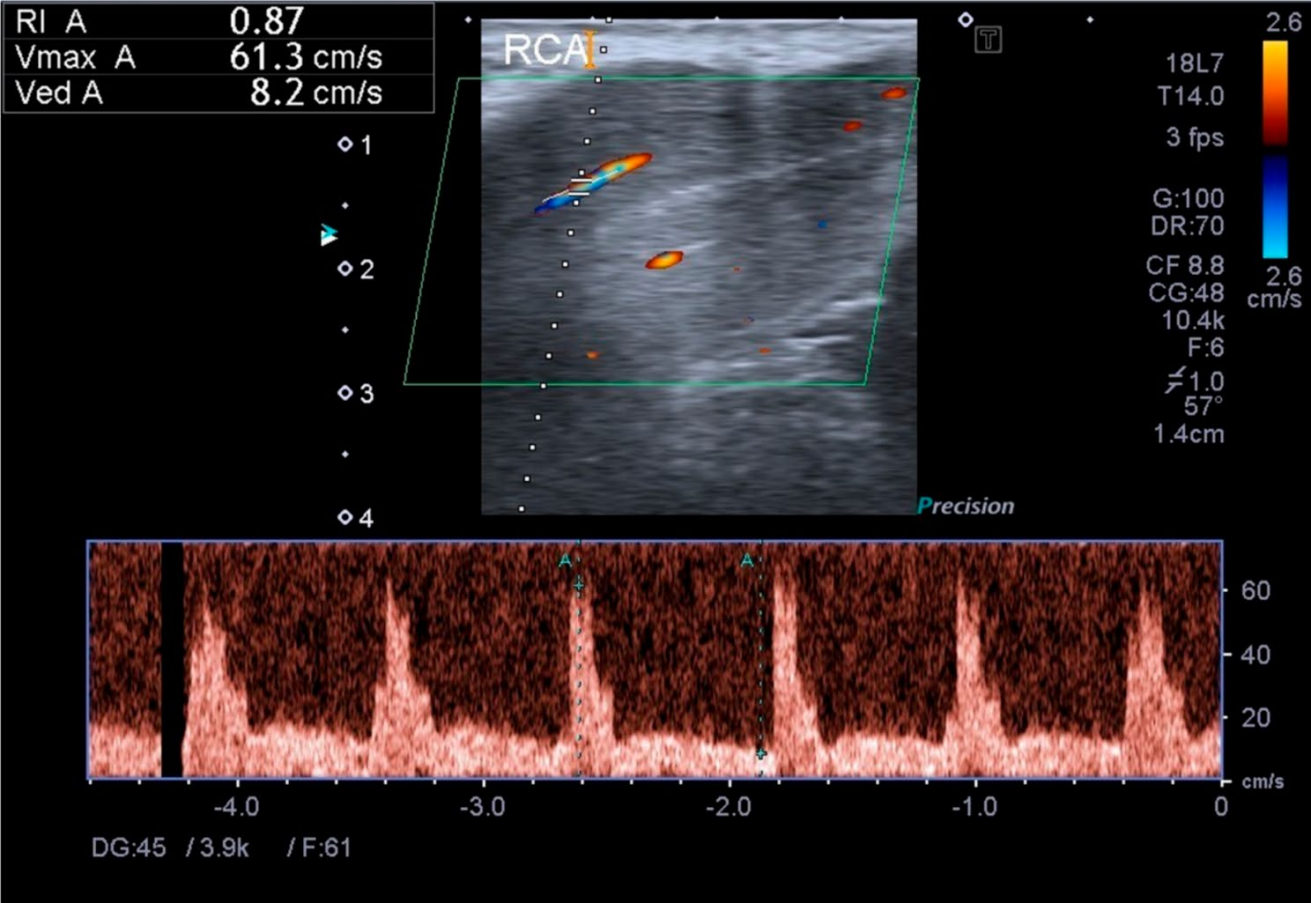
Doppler ultrasound Images of vascular flow in penis
